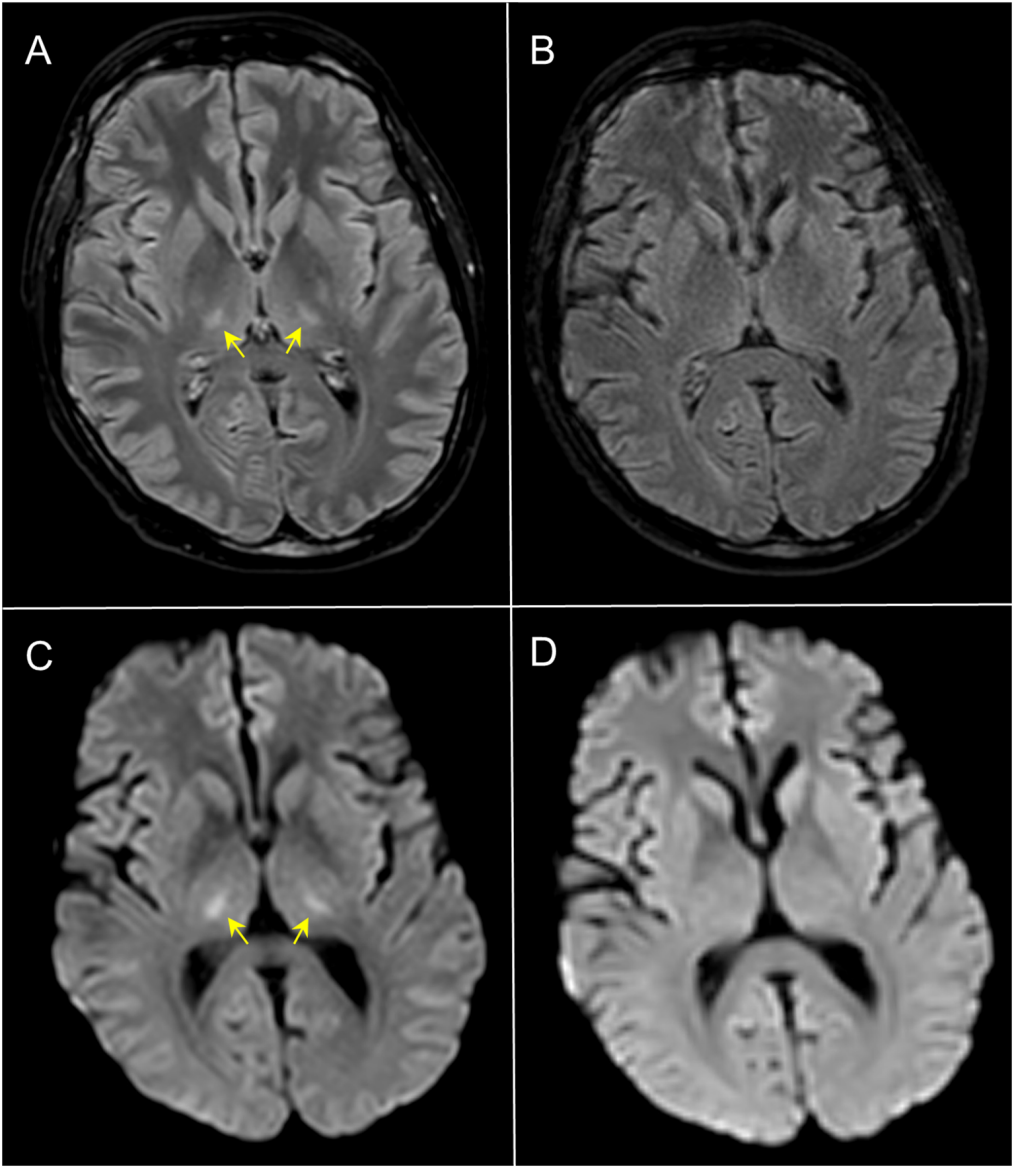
Axial 3D-FLAIR (A, B) and DWI (C, D) MRI. At day +13, 3D-FLAIR showed symmetrical faint hyperintensity of the thalami with corresponding restricted diffusivity (yellow arrows in (A, C) consistent with ICANS; follow-up imaging at day +23 (B, D) demonstrated complete normalization of the signal abnormalities.
